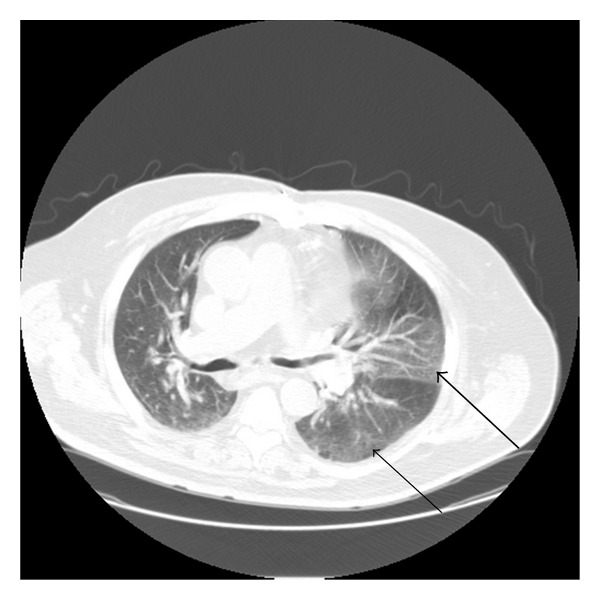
Admission chest computed tomogram with intravenous contrast revealing new ground glass infiltrates of the upper (bold arrow) and lower (narrow arrow) lobes of the left lung.
